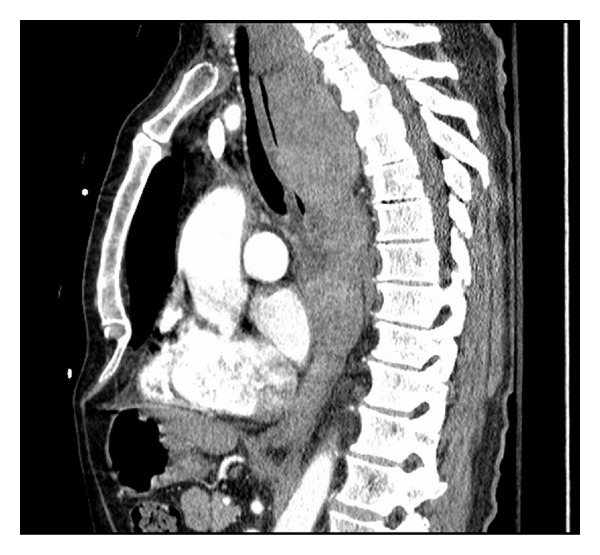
Sagittal view of a Posterior Mediastinal Hematoma measuring 5.9 cm × 5.8 cm × 21 cm, identified via CT scan upon patient presentation to MGH.
